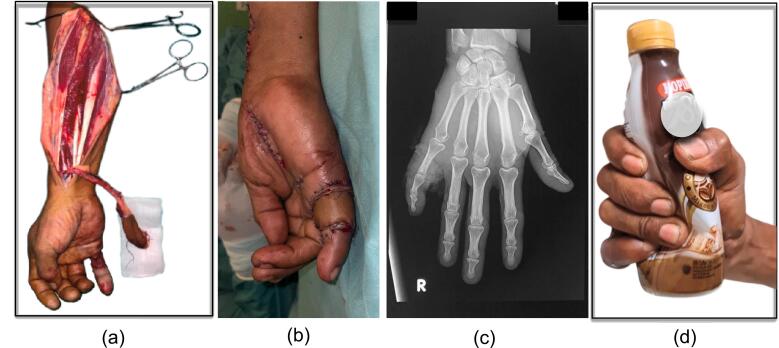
After Chinese forearm flap (a) flap harvesting (b) flap application on wound defect (c) X-ray 3 months after CFA (d) hand function 3 months after CFA.
